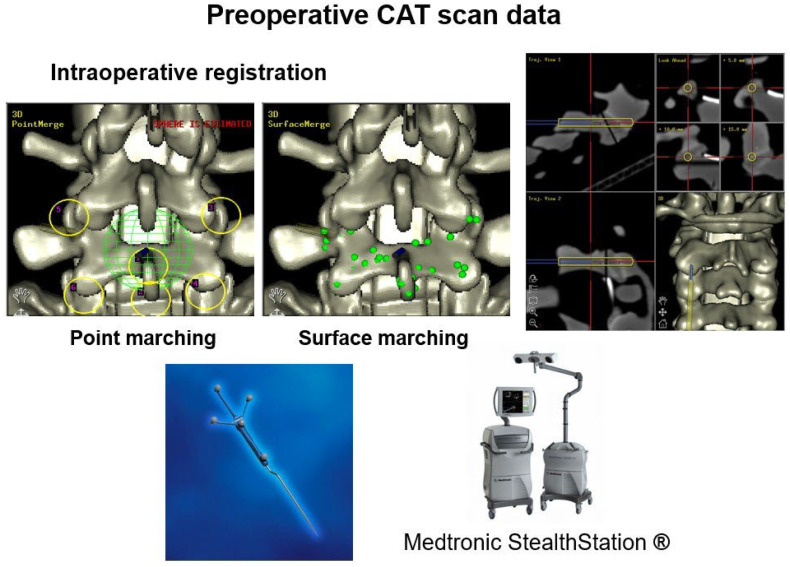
Images demonstrating preoperative CT scan data with different types of registration techniques in preoperative CT-based navigation.
